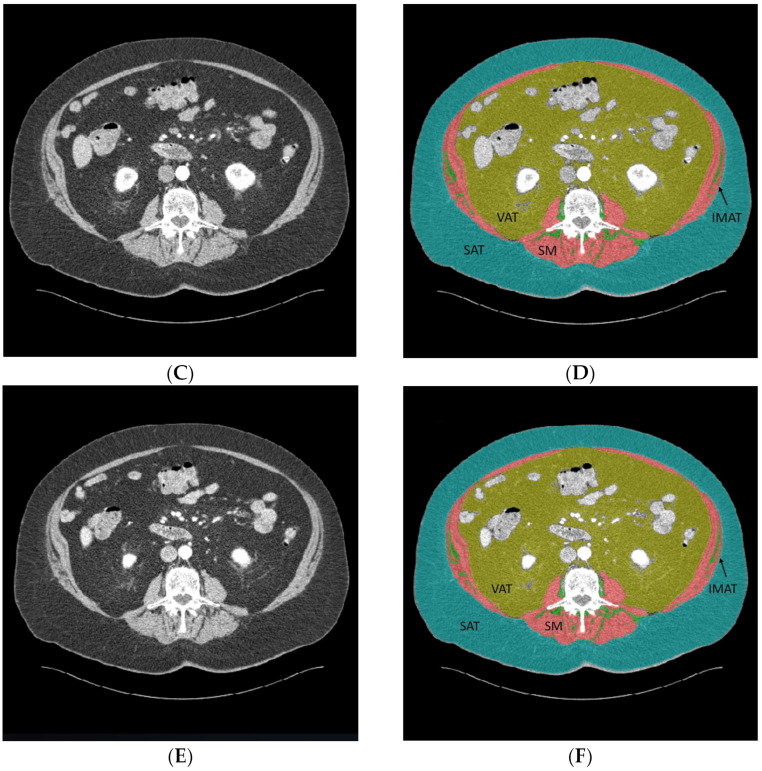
(A) Noncontrast CT and (B) DAFS v3 segmented noncontrast CT at the axial mid-L3 level. In each segmented image, red segmentation represents the skeletal muscle (SM) area. Teal segmentation represents subcutaneous adipose tissue (SAT). Olive green represents visceral adipose tissue (VAT). Bright green represents intermuscular adipose tissue (IMAT; corresponds to tissue designated by the black arrows among skeletal muscle). (C) Arterial phase post-contrast CT and (D) segmented arterial CT at the same level. (E) Venous phase post-contrast CT and (F) segmented venous CT at the same level.
